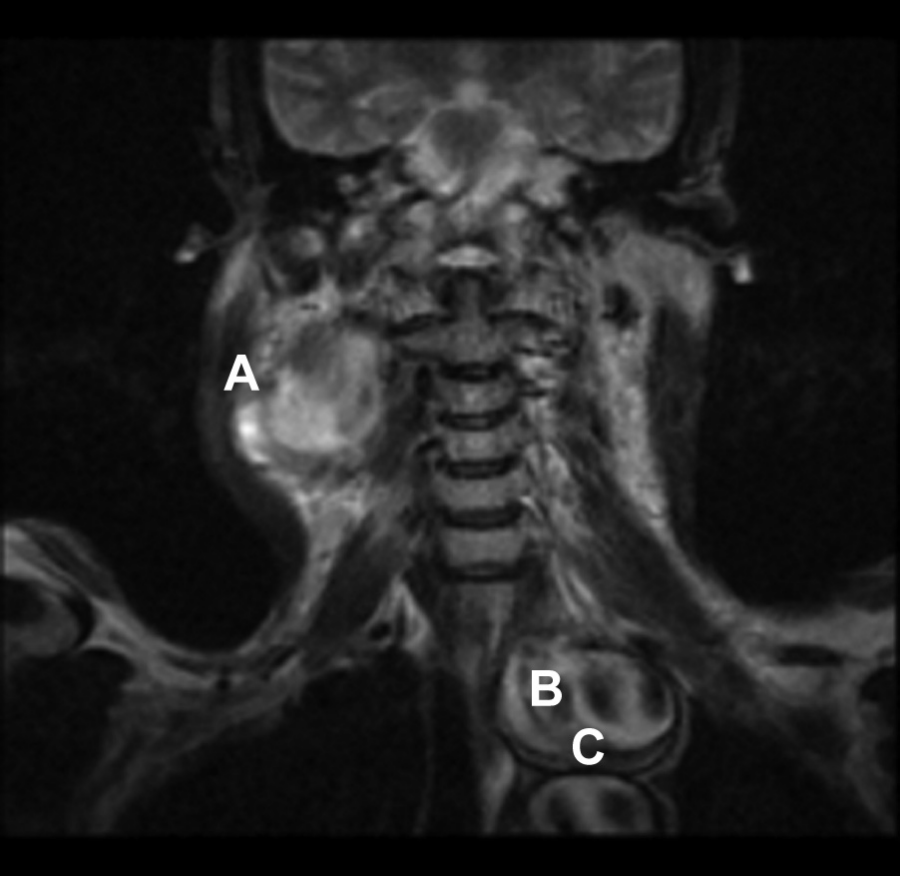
Noncontrast MRI scan of neck and upper thorax showing (A) right common carotid artery pseudoaneurysm, (B) proximal left subclavian artery pseudoaneurysm, and (C) pseudoaneurysm at the origin of the left subclavian artery.
